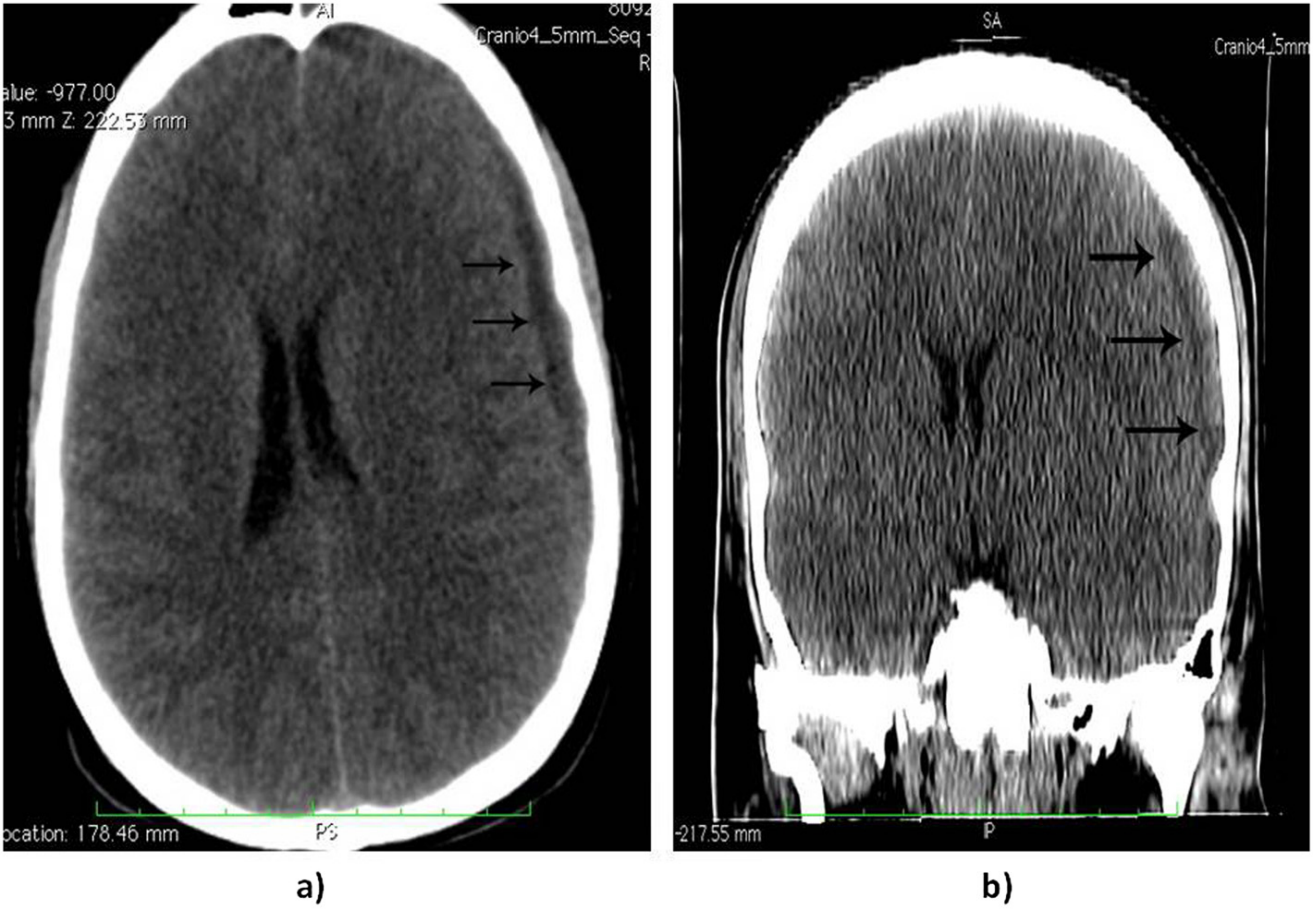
Computed tomography scan in axial (a) and coronal (b) cut: displaying the subdural collection (black arrows) of exudates on the left side in the temporal-parietal region. The air content of the empyema is noticeable.
